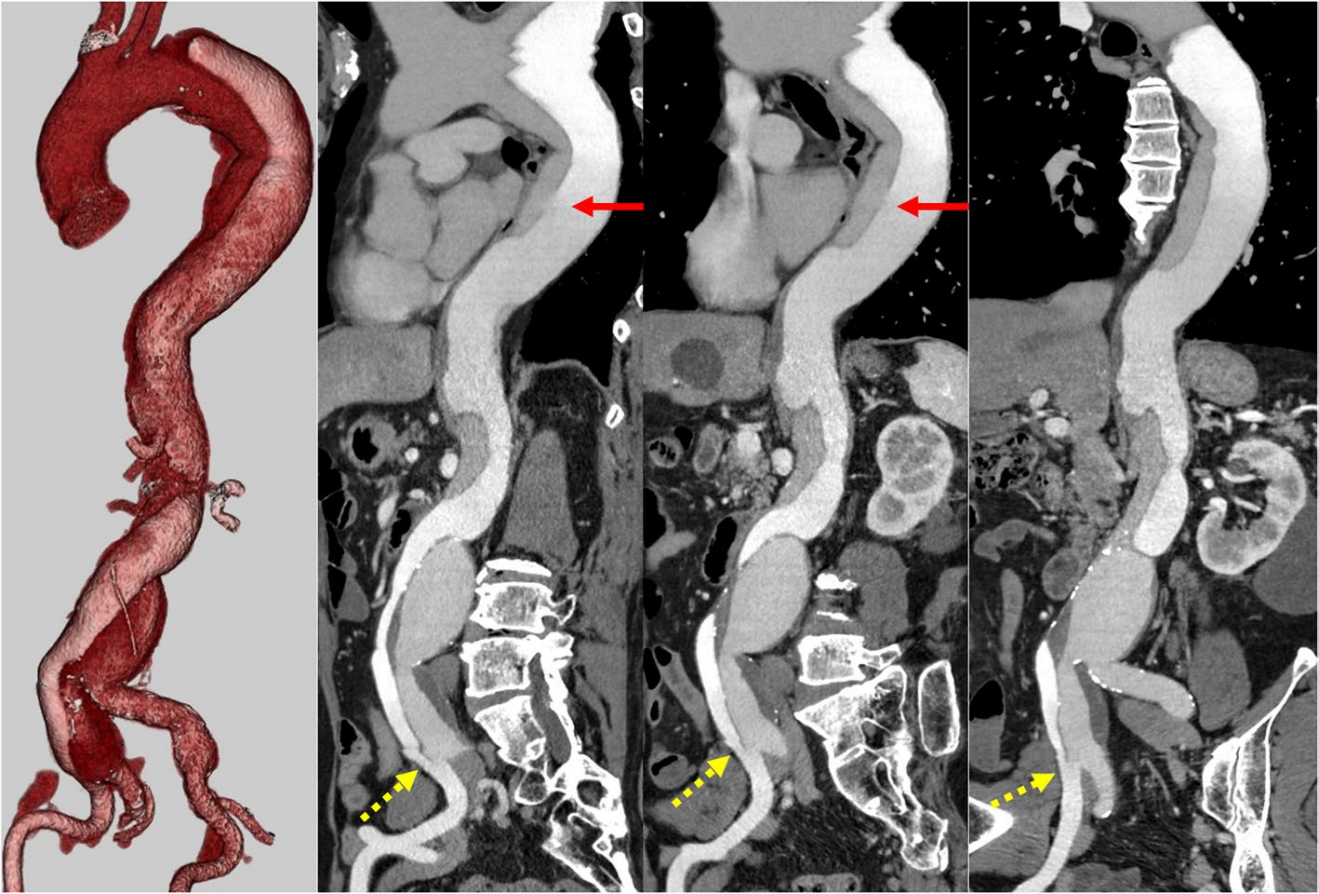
Preoperative contrast-enhanced CT (3-dimensional and stretch curved planar reconstruction). CT reveals type B aortic dissection and a thoracoabdominal aortic aneurysm, an abdominal aortic aneurysm, and a right common iliac artery aneurysm. Red arrows indicate the proximal tears. Yellow dotted arrows represent distal tears. CT, computed tomography
